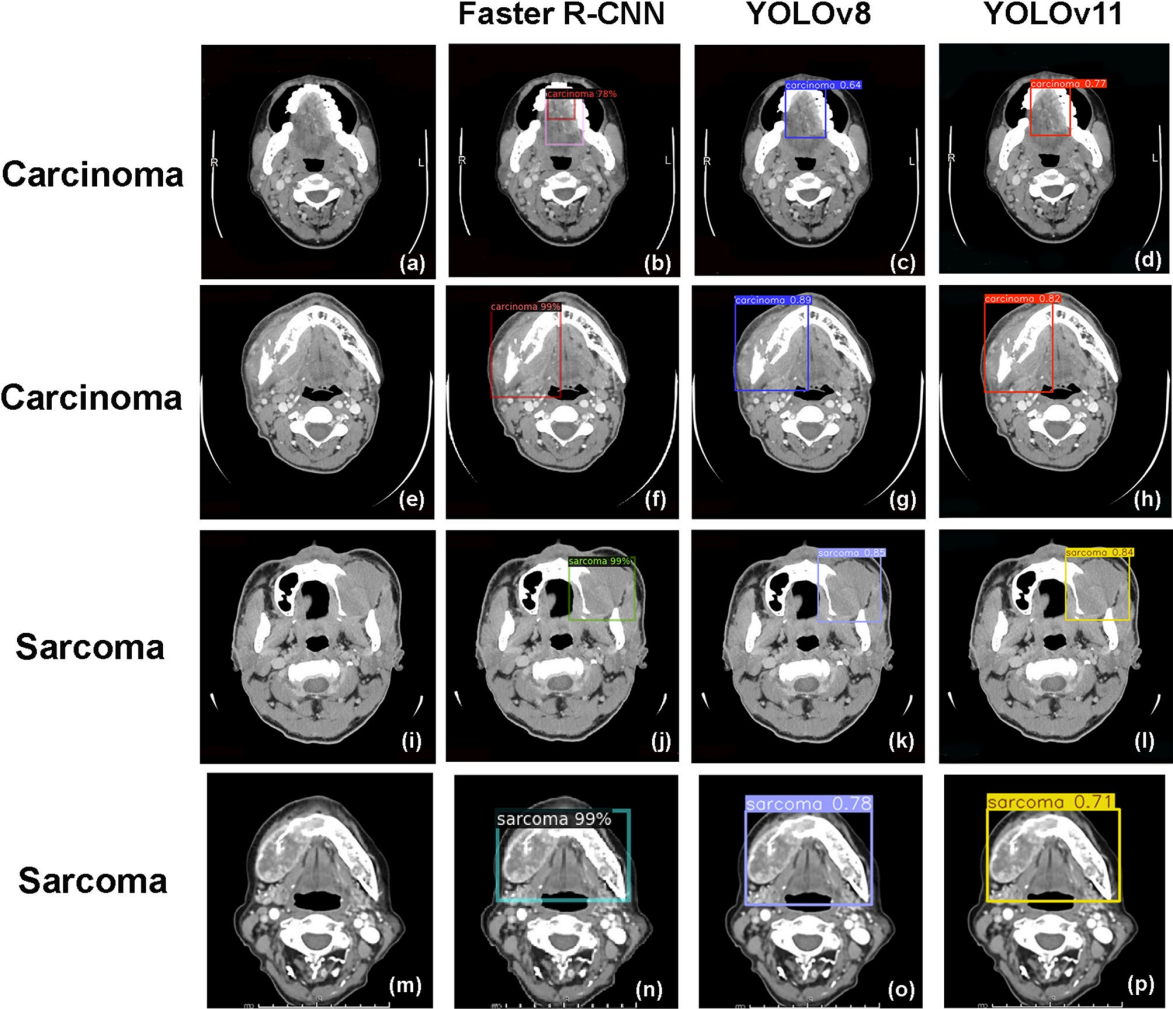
Examples of original CT images with detection bounding boxes generated by Faster R-CNN, YOLOv8, and YOLOv11 for detecting oral carcinoma and sarcoma from the test set. True positive outputs for the carcinoma class from Faster R-CNN, YOLOv8, and YOLOv11 in patients with oral squamous cell carcinoma had intersection over union (IoU) values of 0.78, 0.99, and 0.64, and 0.89, and 0.77, 0.82 respectively (b,c,d,f,g,h). True positive outputs for the sarcoma class from Faster R-CNN, YOLOv8, and YOLOv11 in patients with B-cell lymphoma had IoU values of 0.99, 0.85, and 0.99, and 0.78, and 0.84, 0.71 respectively (j,k,l,n,o,p). IoU Intersection over union.
